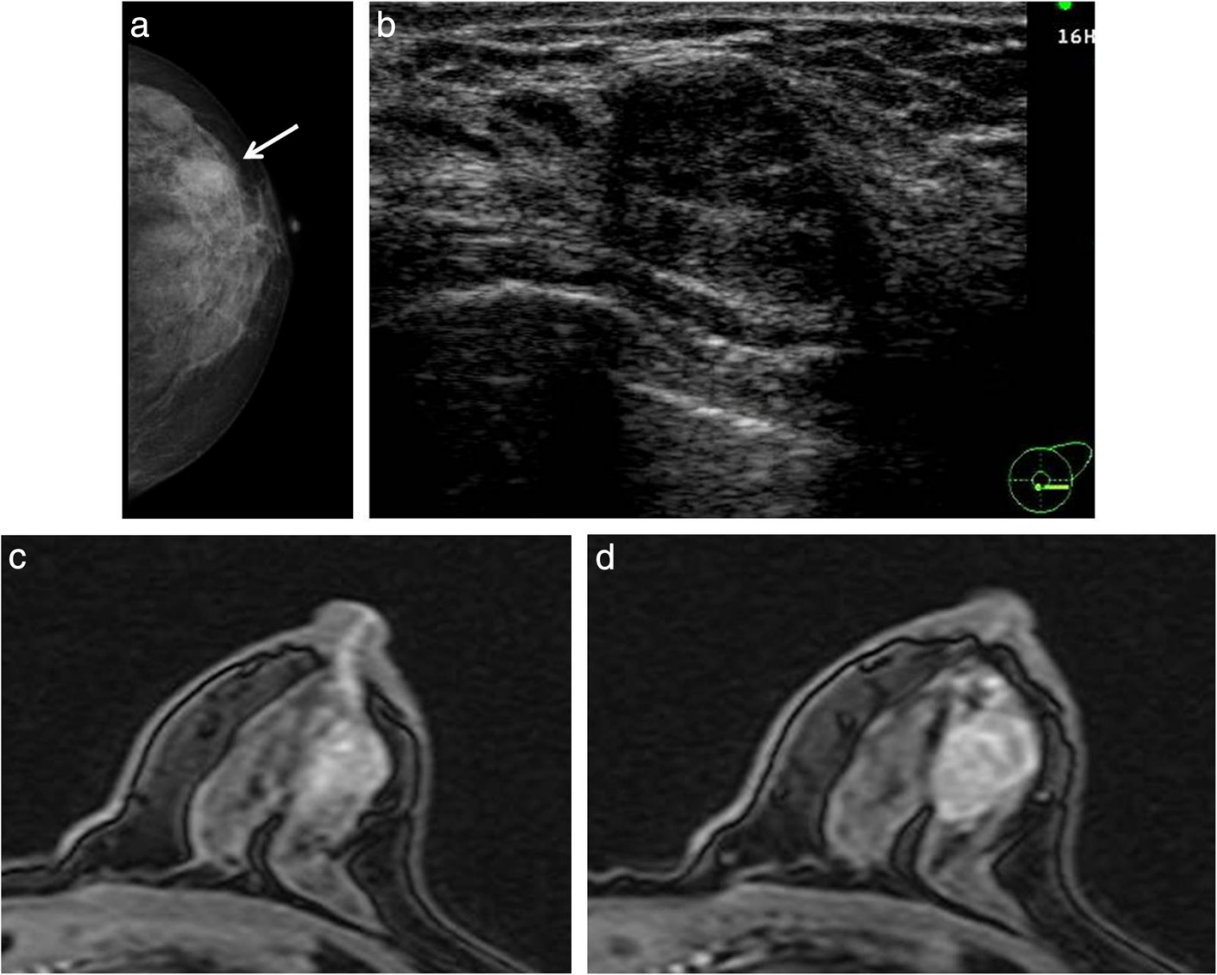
a Mammography showed an irregular oval mass (*arrow*) in the outer quadrant of the left breast. b Ultrasonography showed a well-circumscribed hypoechoic mass. c MRI showed high intensity from the mass to the nipple. d Enhanced MRI revealed a mass with an early peak and a delayed washout pattern and ring enhancement
